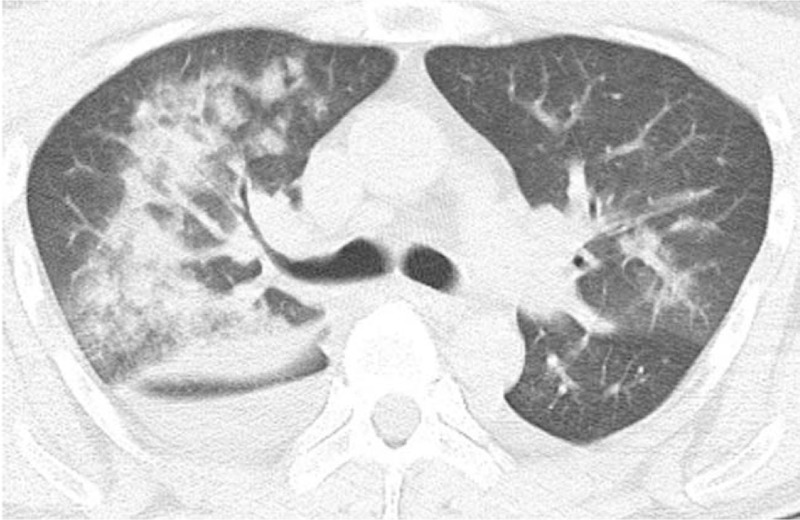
Computed tomographic image when the patient initially reported to our hospital. Exudation in both lungs, which is more significant on the right side, and a small amount of bilateral pleural effusion is observed.
